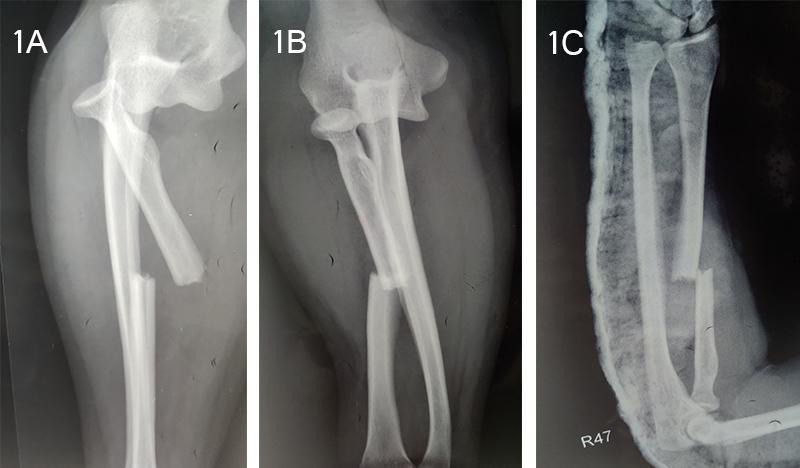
A, B, C. Plain radiographs showing radial head dislocation with ipsilateral radial shaft fracture (A) Lateral view (B) AP view (C) Immediate postoperative Xray following closed reduction of the radial head dislocation.
